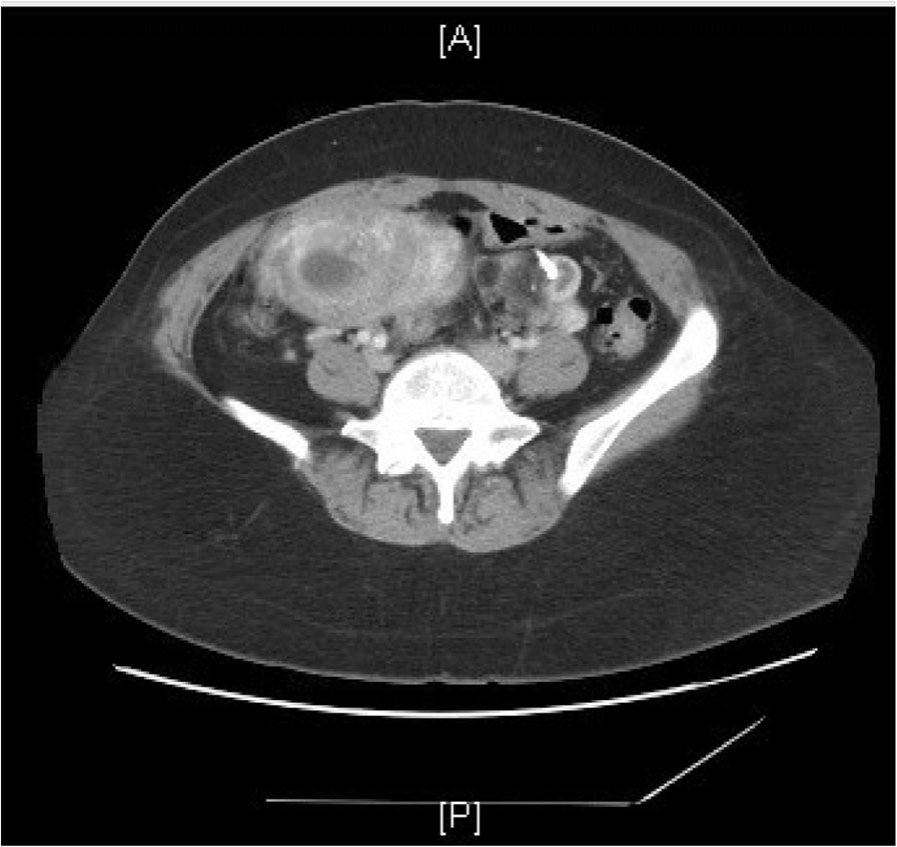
Axial view – Abdominal CT scan showing a left sided ovarian teratoma with calcification
